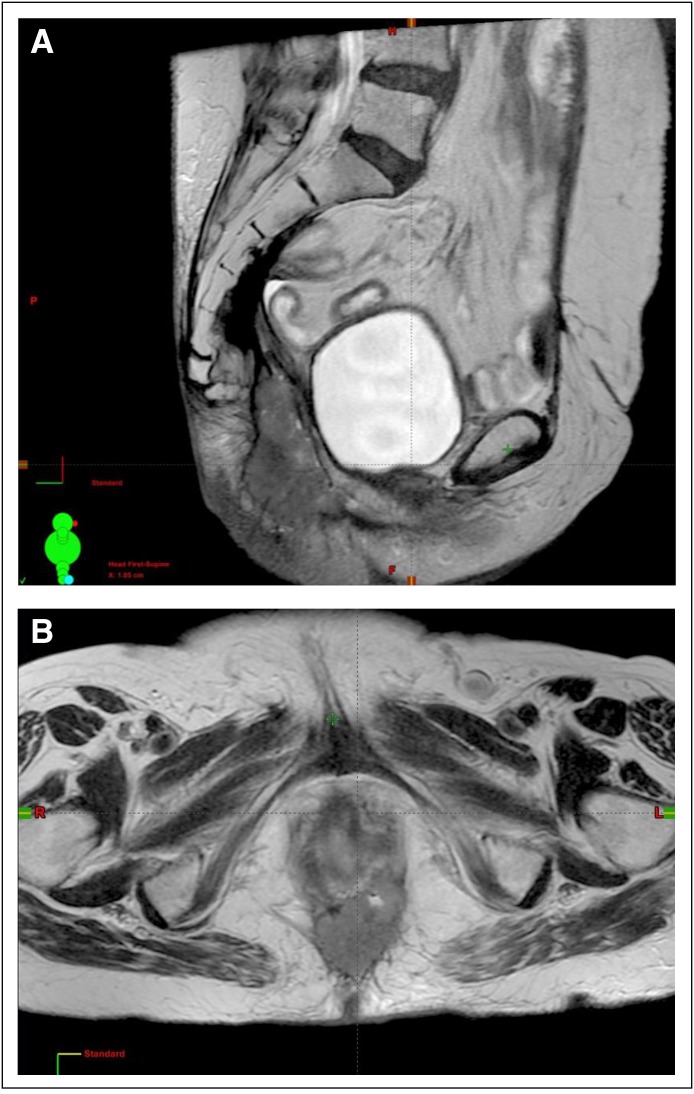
(A) Sagittal T2-weighted magnetic resonance image of the pelvis showing expansive 5.6 cm lesion at rectal topography. (B) Axial T2-weighted magnetic resonance image of the pelvis showing expansive lesion at rectal topography.
